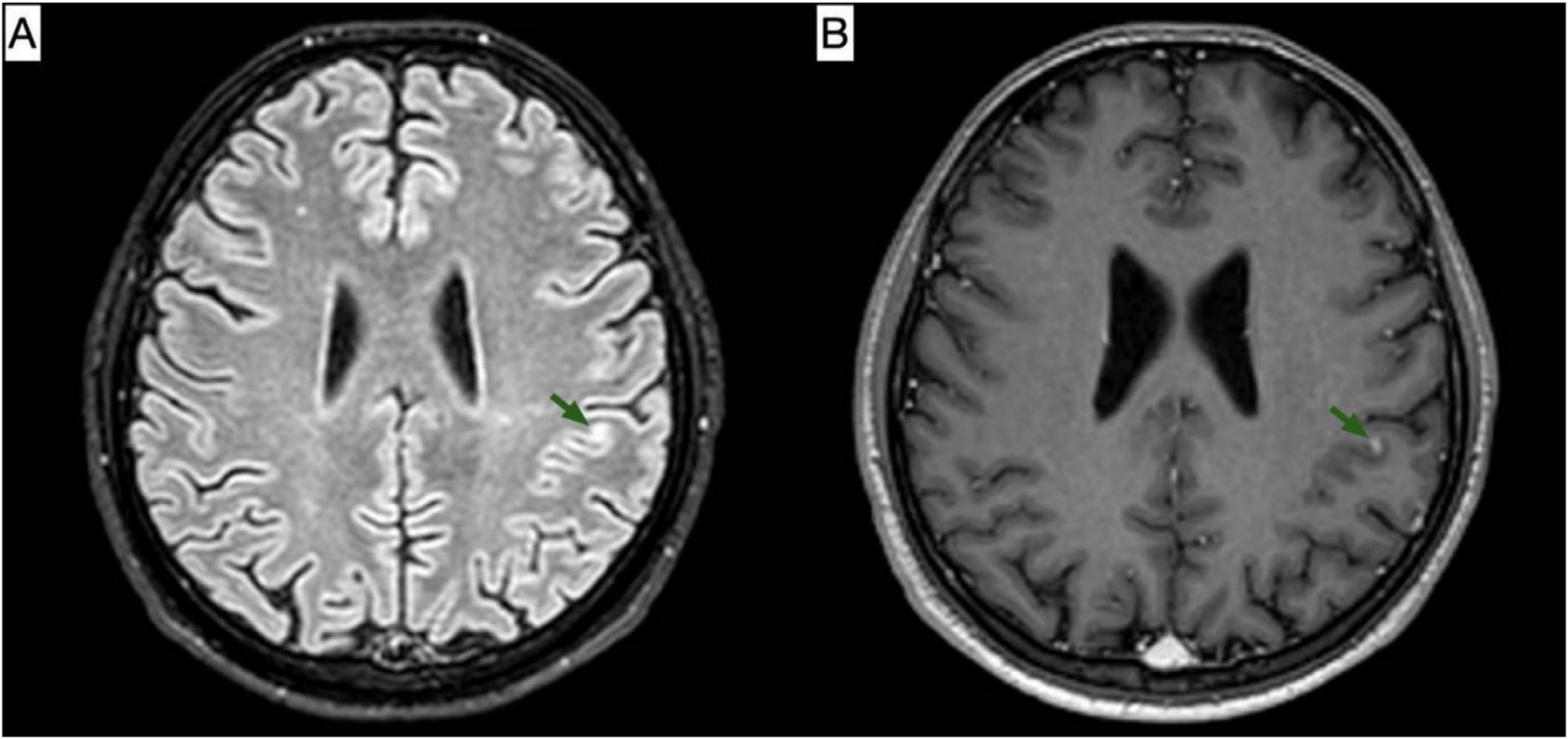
(A) FLAIR sequence; (B) T1-weighted image post-contrast. Selected axial MRI images demonstrate an enhancing focus at the left parietal corticomedullary junction, which appears hyperintense on the FLAIR sequence (indicated by green arrows), suggestive of a metastatic focus.
